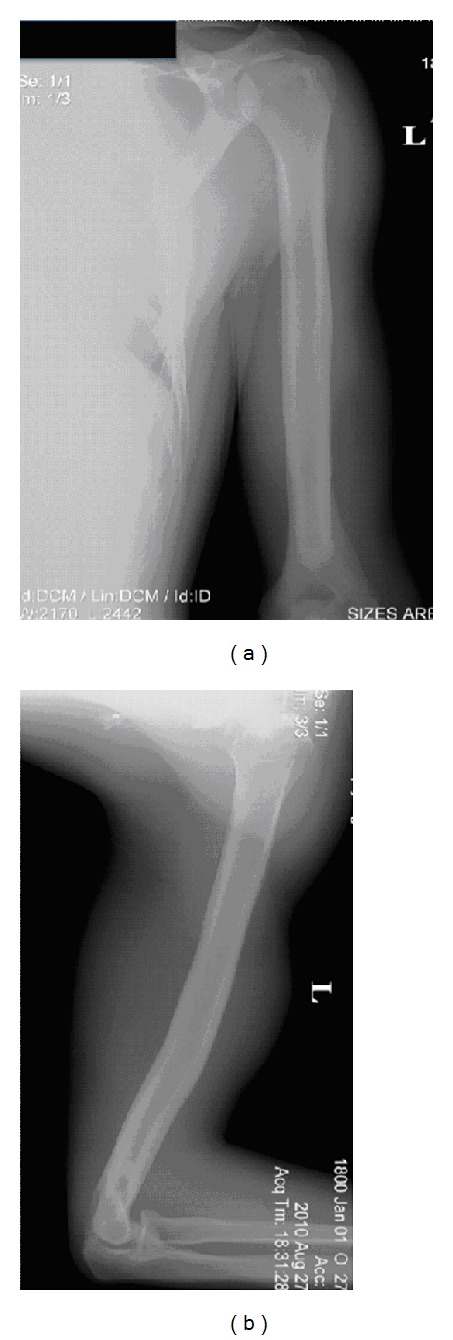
Anterior-posterior and lateral radiographs of the left humeral shaft at 10-year followup. The patient has near anatomic alignment in the coronal plane and a 10-degree anterior bow in the sagittal plane.
